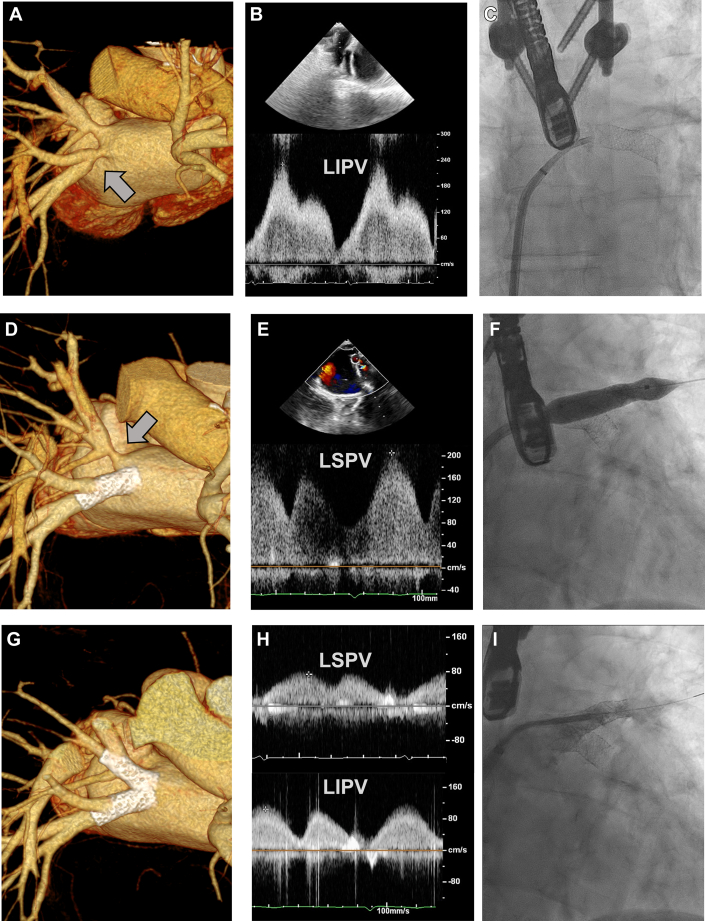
Staged Percutaneous Reconstruction of Iatrogenic Pulmonary Vein Stenosis (A) Computed tomography angiography showing severe left inferior pulmonary vein stenosis (LIPV, arrow). (B) Doppler echocardiography demonstrating elevated flow velocity in the left inferior pulmonary vein. (C) Fluoroscopic view after stent implantation in the inferior vein. (D) Computed tomography angiography showing progression of left superior pulmonary vein stenosis (LSPV, arrow). (E) Doppler velocities before superior vein stenting. (F) Deployment of superior vein stent. (G and H) Follow-up computed tomography and Doppler confirming patency of both stents. (I) Final fluoroscopic image showing 2 stents in the left pulmonary veins.
